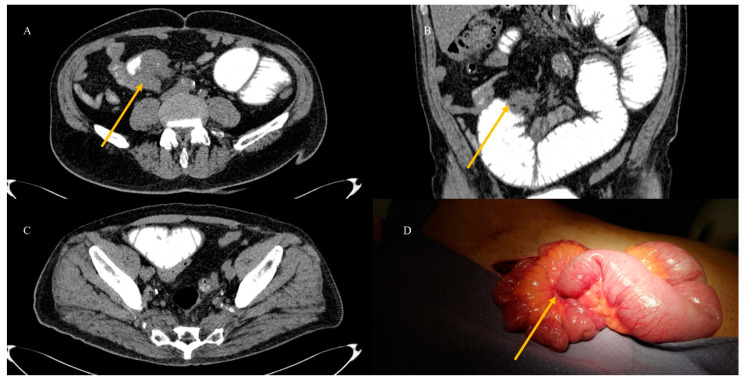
This figure shows a CT scan with GGF, which confirms the irregular stenotic thickness of a distal jejunal loop (orange arrow) in image (A). In image (B), irregular tissue in the mesenteric fat (orange arrow) is visible, along with a high grade of obstruction seen in both images (B,C) where GGF transit is stopped. Prompt surgical intervention confirmed the neoplastic stenosis (orange arrow) in image (D), resulting in an invasive intestinal adenocarcinoma.
